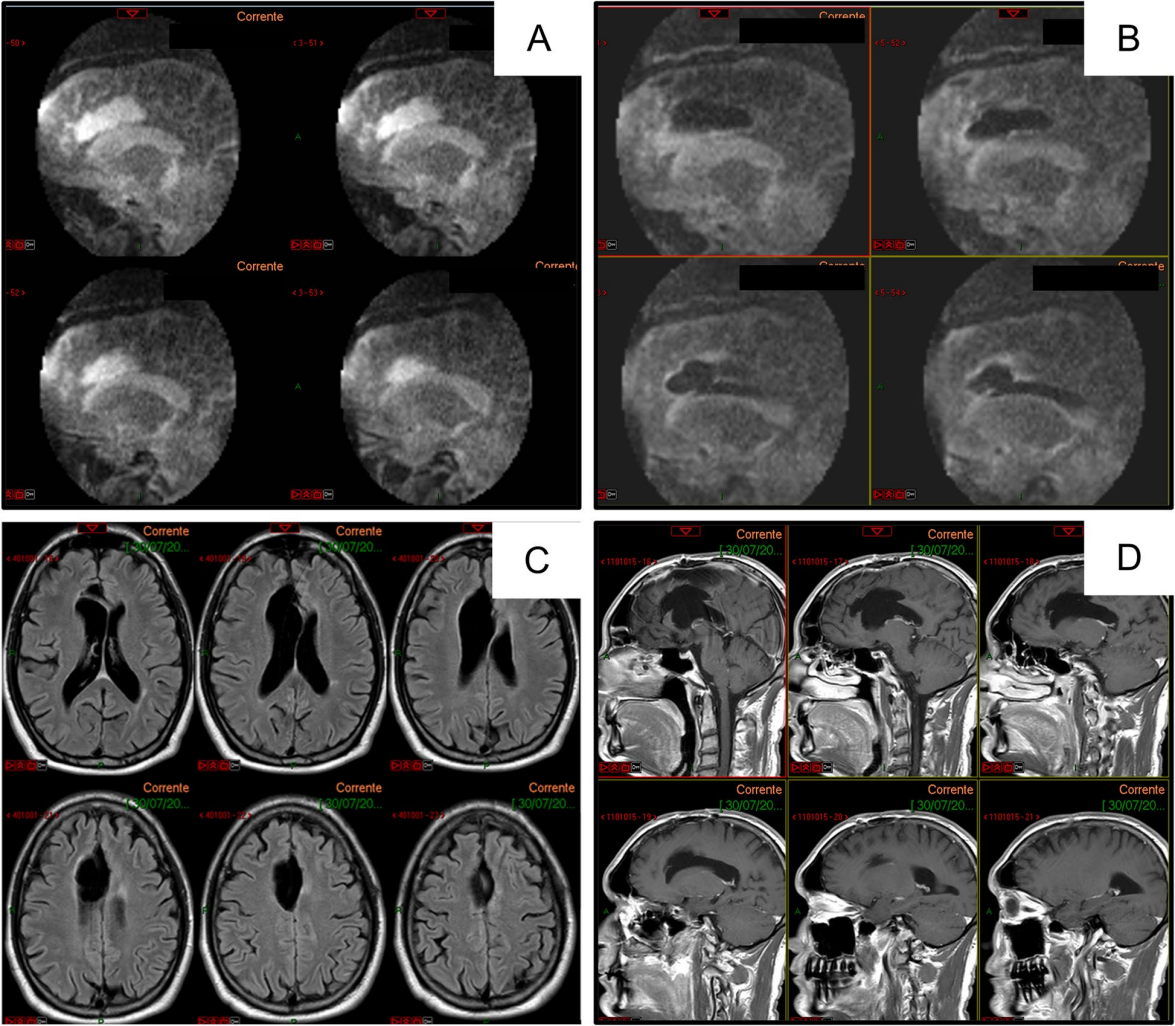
Intraoperative MRI scan showing complete removal of the lesion, as demonstrated comparing the T2-weighted sequences before (A) and after (B) surgery; three months later FLAIR (C) and contrast enhanced T1-weighted (D) magnetic resonance images showing no recurrence
